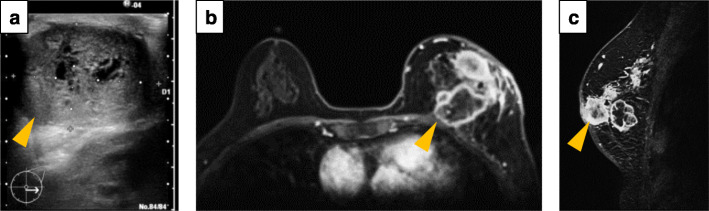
The data of the secondary operation. a An ultrasonography (US) scan of the breast demonstrated a scale out size of low echoic mass (orange arrowhead). b A magnetic resonance imaging (MRI) showed some tumors including internal necrosis with ductal spread (orange arrowhead). The pectoralis muscle invasion was suspected. c A sagittal sequence of MRI scans showed the wide ductal spread of malignant adenomyoepithelioma (AME), which invaded the skin (orange arrowhead)
